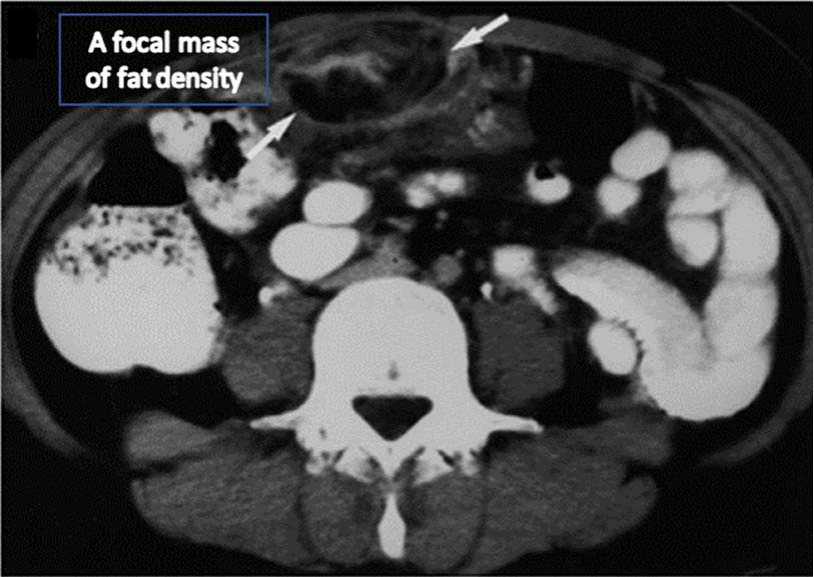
Computed tomography section of the abdomen showing a focal mass of fat density (indicated with white arrows).
